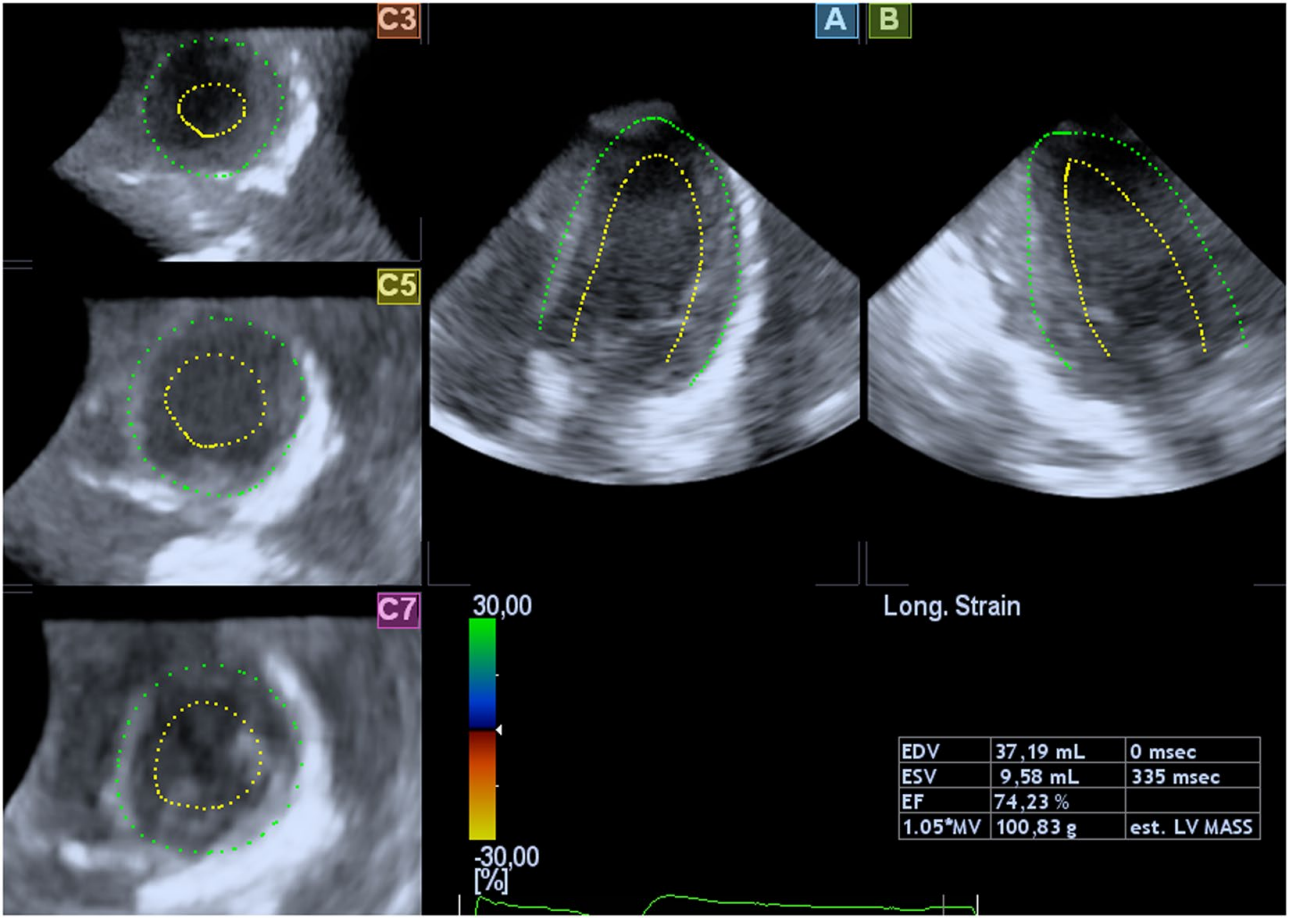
Sample images of the heart by RT3DE (software Toshiba). Two-dimensional slices from a representative real-time 3D echocardiographic data set with endocardial (yellow) and epicardial (green) borders of the left ventricular myocardium.
